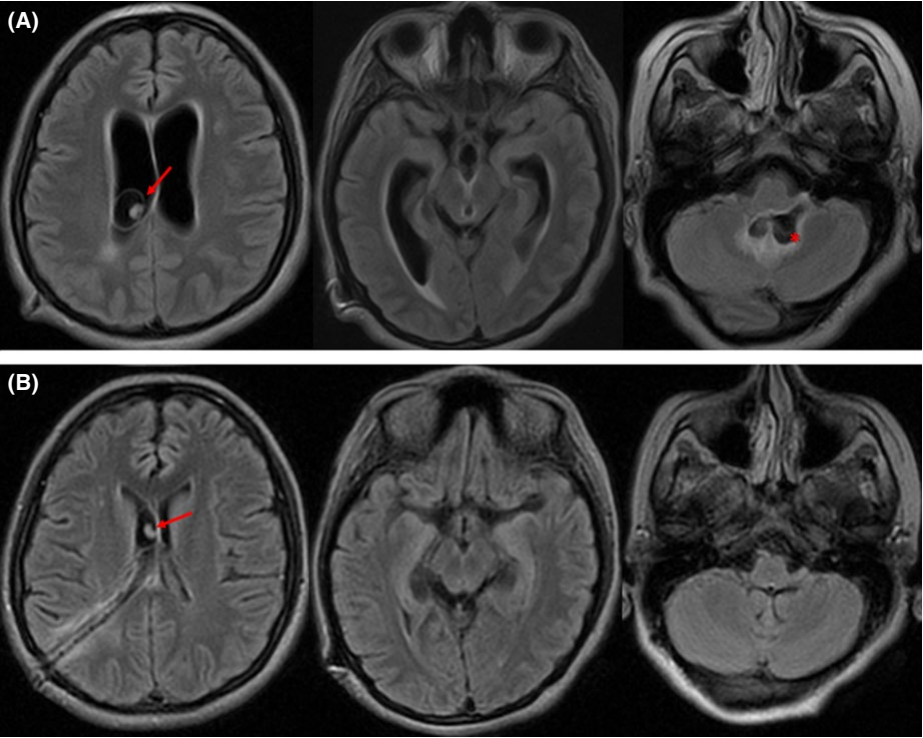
MRI FLAIR sequence. A, Pretreatment images demonstrate communicating hydrocephalus with transependymal CSF egress. Note the intraventricular cyst with scolex (arrow) in the right lateral ventricle, and another cyst in the fourth ventricle (asterisk). B, Post‐treatment images, obtained 3 weeks later, show resolution of hydrocephalus and fourth ventricle cyst
